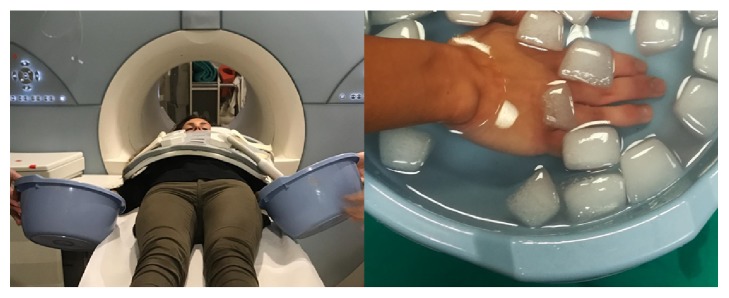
Cold pressor test execution: both hands are completely immersed in ice water for four minutes. Only after the end of the 4 minutes was the perfusion MRI performed.
